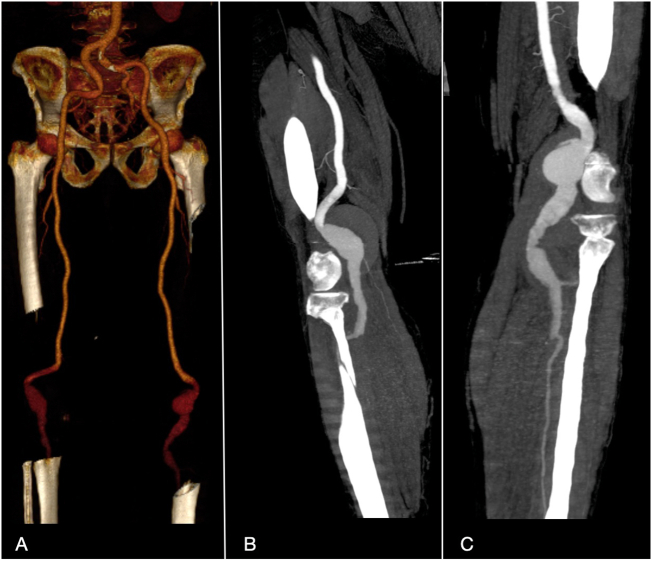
CT angiography 3-dimensional volume rendering showing bilateral popliteal aneurisms (A), and MPR right (B) and left (C). CT angiography before the first procedure.
